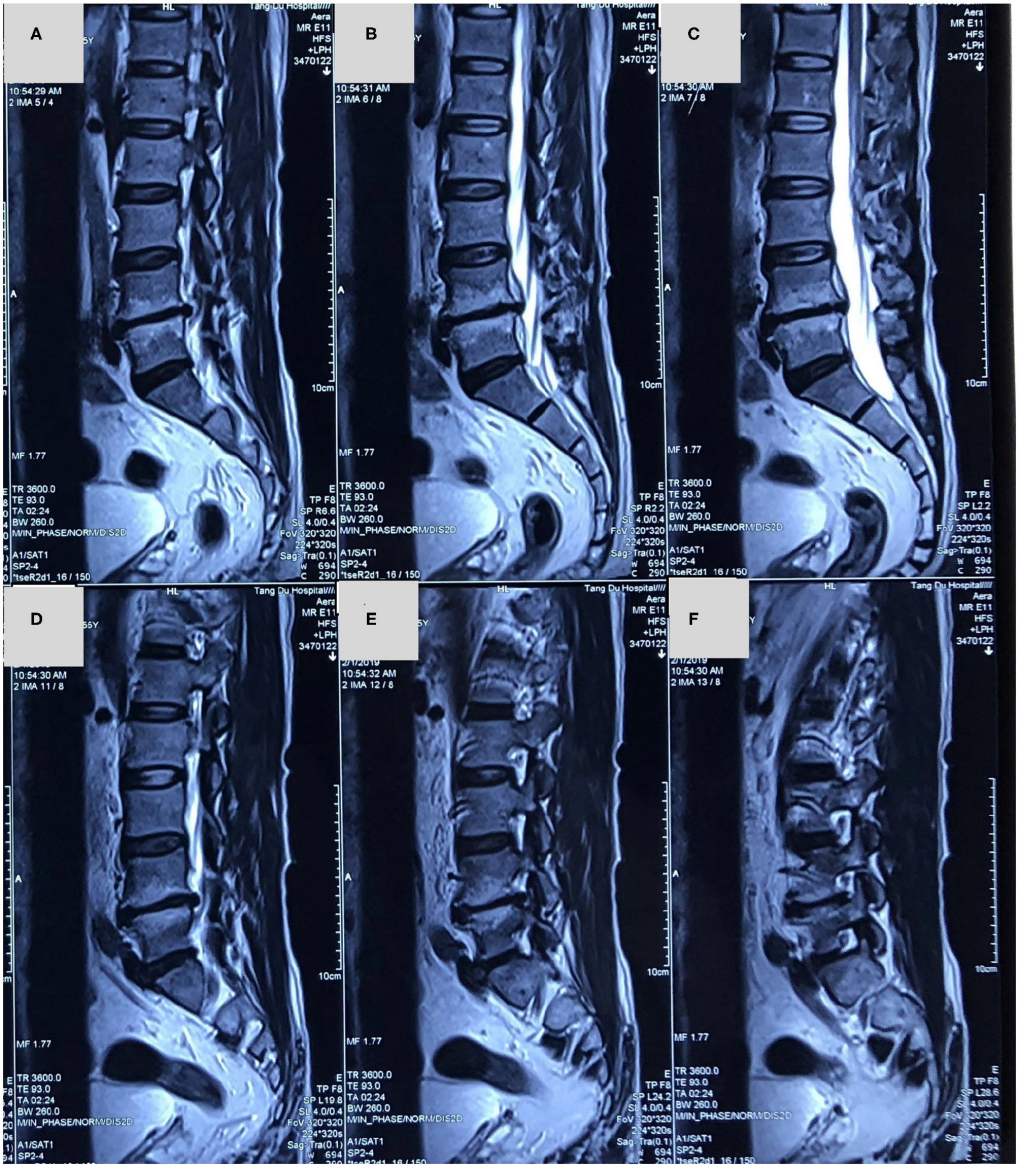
(A–F) A magnetic resonance imaging in sagittal slices showed that lumbar disc herniation in L4/5 segment and L4/5 intervertebral disc was in a condition of Modic II type degeneration.
